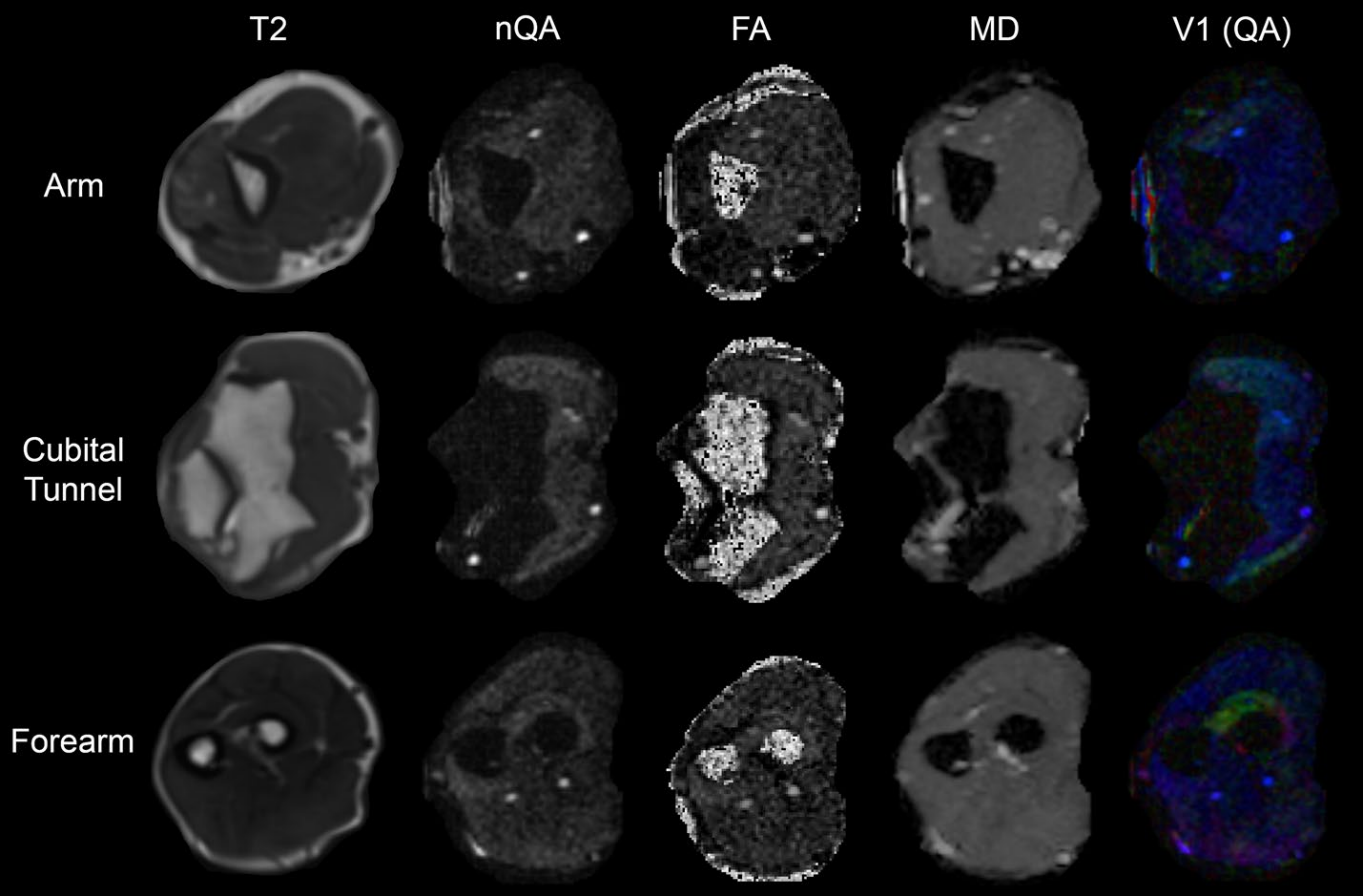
Data derived from a healthy control. The rows show data from the arm, cubital tunnel and forearm. The columns contain T2-weighted scans, and corresponding maps of normalised quantitative anisotropy (nQA), fractional anisotropy (FA), mean diffusivity (MD) and the principal eigenvector (v1) with the colours red, green and blue representing diffusion in x, y and z directions, and the intensity scaled by quantitative anisotropy (QA).
